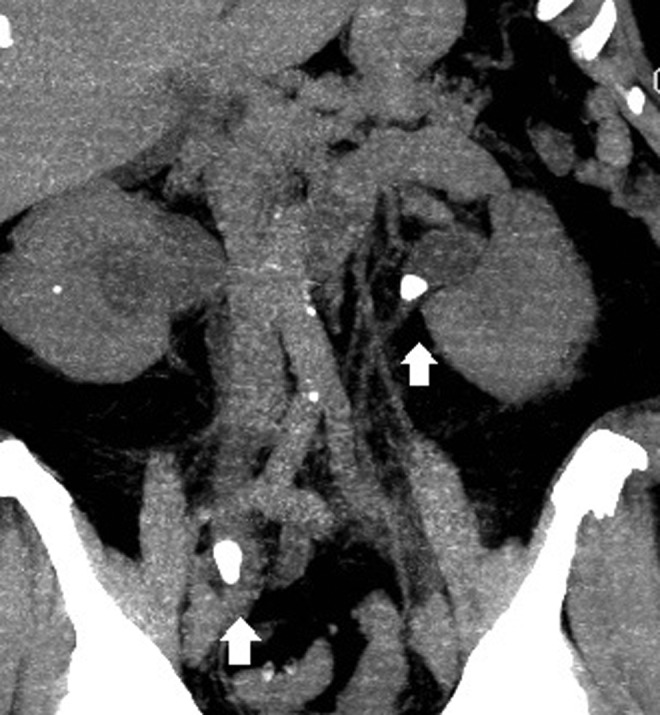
Noncontrast CT scan with 1.5 cm stone in right distal ureter and 1.1 cm stone at left ureteropelvic junction with associated hydronephrosis.
